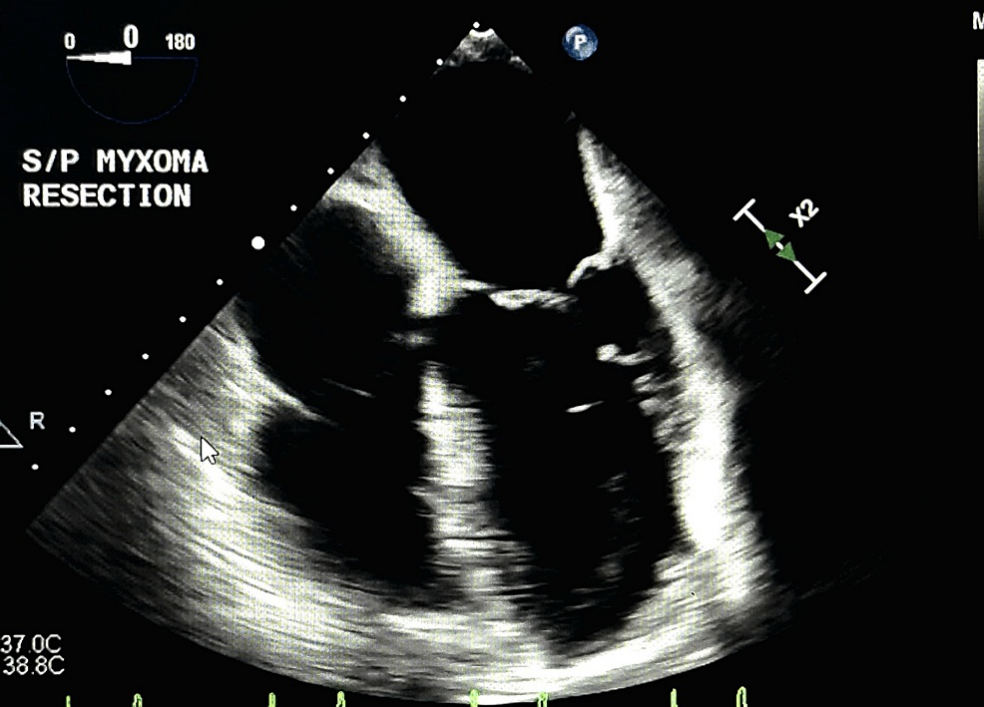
Echocardiogram showing a four-chamber view post-resection of myxoma.
